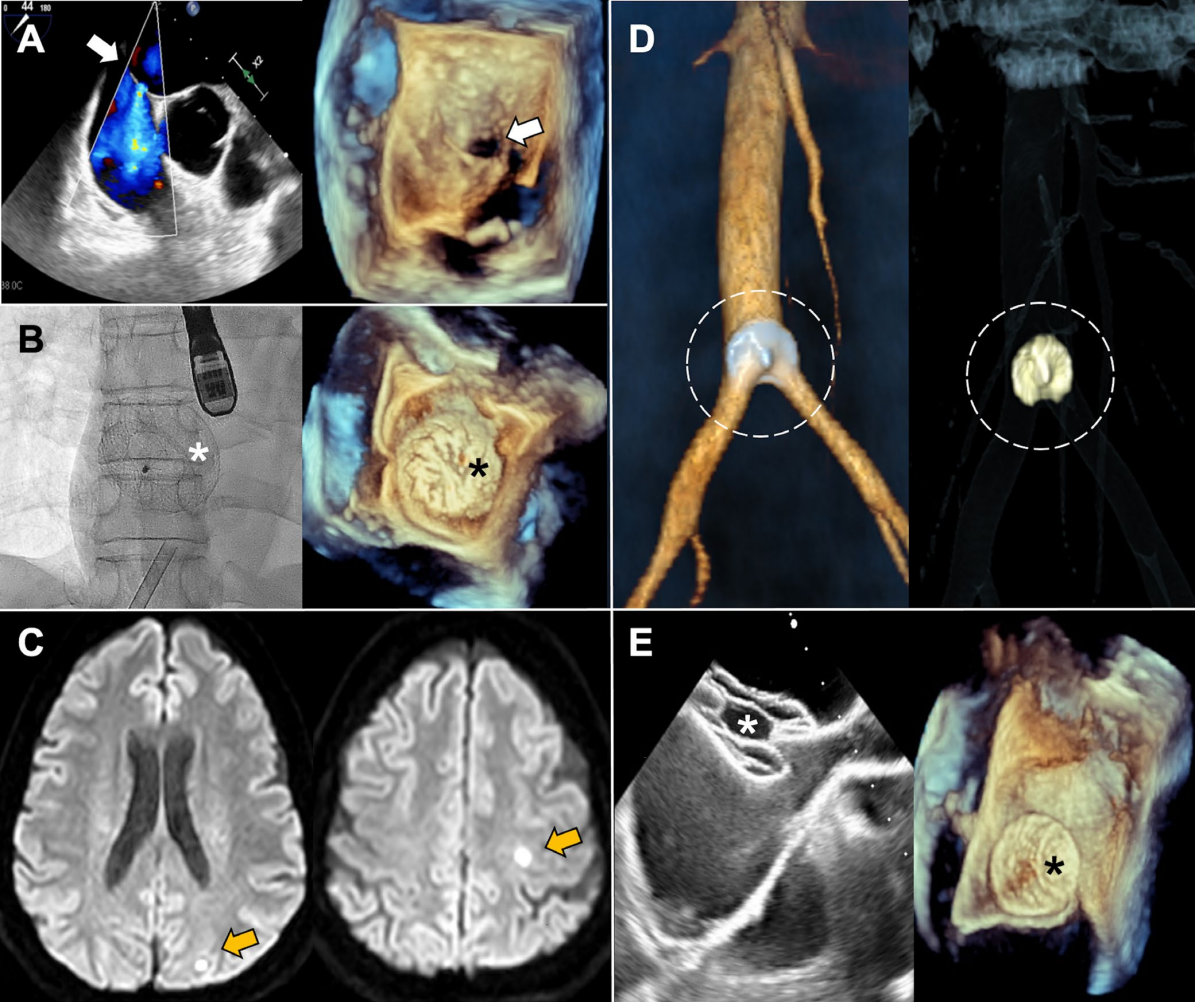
(A) Left‐to‐right shunt across an ASD‐OS seen on the TEE color Doppler (white arrow, left) and 3D‐TEE (white arrow, right). (B) First device implantation: 17 mm Figulla Flex II UNI seen by fluoroscopy (white asterisk) and 3D‐TEE (black asterisk). (C) Brain MRI showing two acute lacunar infarcts with restricted diffusion (orange arrows) in the left occipital lobe and the precentral gyrus. (D) 3D‐CT reconstruction showing the embolized occluder at the aorto‐iliac bifurcation (dashed circle). (E) Second device implantation: 16 mm Occlutech 29ASD visualized by TEE (white and black asterisks). 3D‐CT, three‐dimensional computed tomography; ASD‐OS, ostium secundum atrial septal defect; MRI, magnetic resonance imaging; TEE, transesophageal echocardiography.
